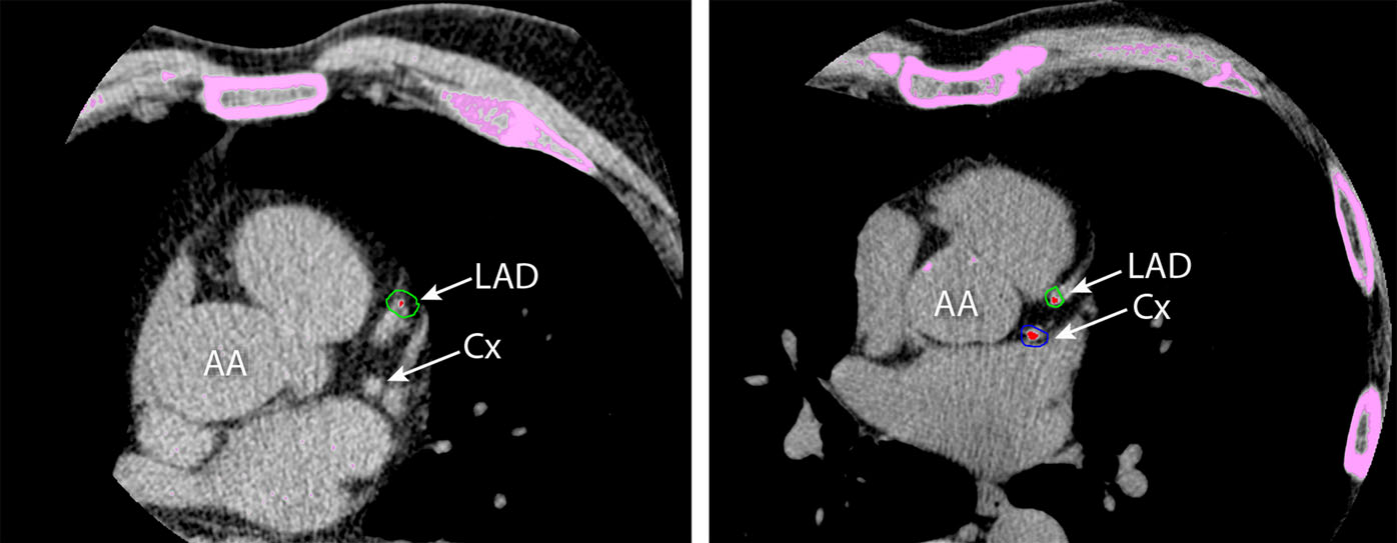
Computed tomography images in axial view showing coronary artery Agatston calcium scores on single slice levels. Total score is summed up for all slices. *Left*, offspring; 68-year-old male, height 1.79 m, weight 89 kg, no risk factors. Total Agatston calcium score was 3, by added scores of 0 in right coronary artery (RCA), 3 in left anterior descending artery (*LAD*), and 0 in circumflex artery (*Cx*). *Right*, control; 64-year-old male, height 1.77 m, weight 82 kg, with risk factor hypertension. Total Agatston calcium score was 68, by added scores of 5 in RCA, 19 in LAD, and 44 in Cx. *AA* ascending aorta, *S* sternum
